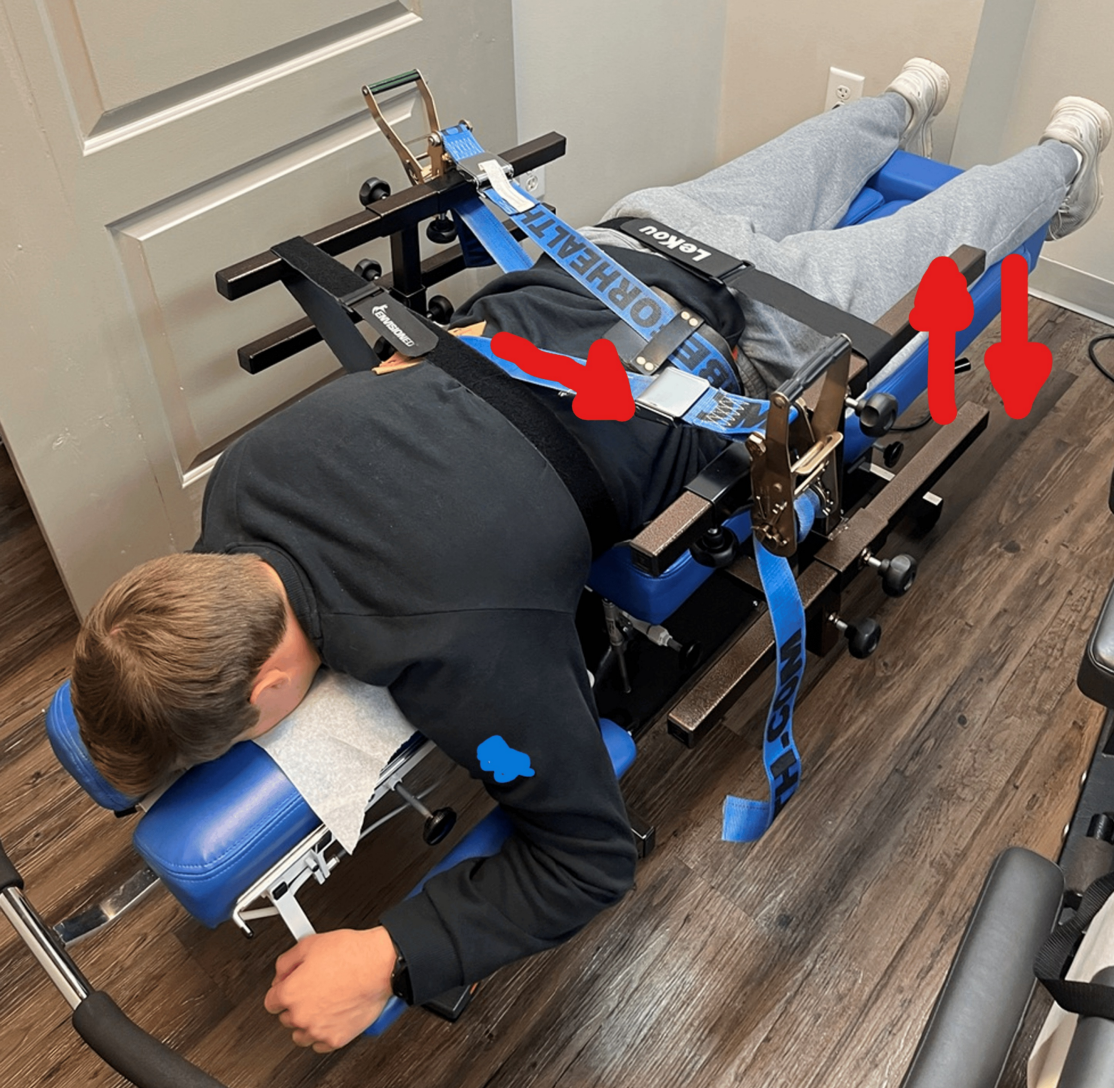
Flexion/distraction table Eckard table (mechanical traction). This is done with mirror image positioning of the patient based on their X-ray configuration. The Eckard table will provide motion and mechanical traction. This therapy will create a range of motion and flexibility, and mechanical traction will be a force used to allow for the separation between joint surfaces. This was performed for 20 minutes each session (model used for demonstration).
